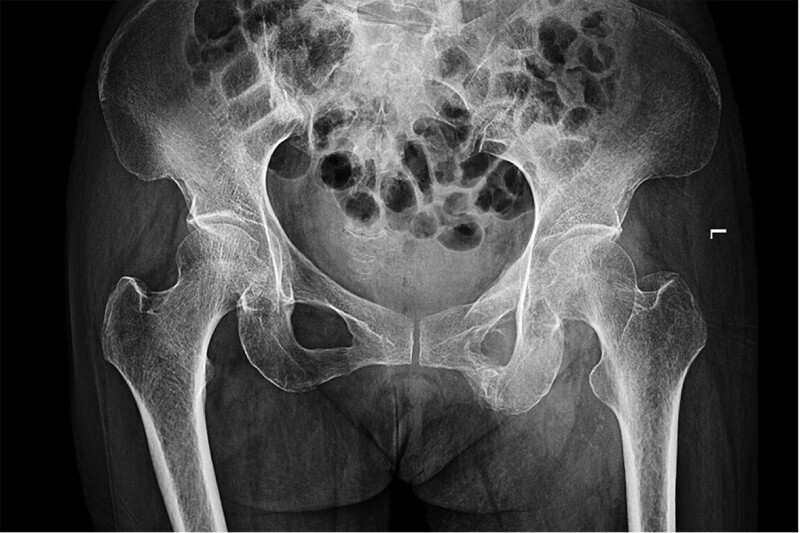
A radiograph of the pelvis taken postoperatively reveals complete resection of the lesion and no residual ossification.
